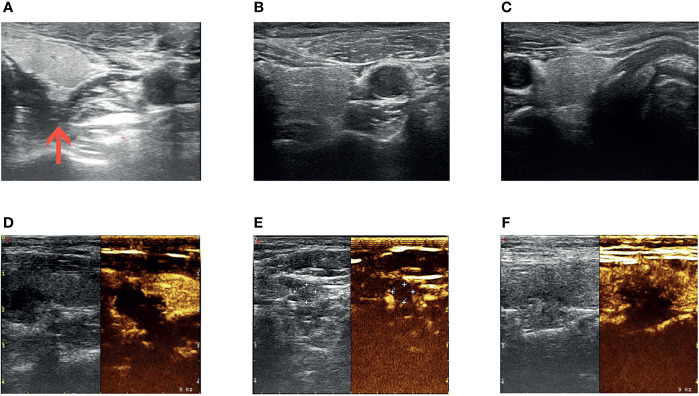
Radiofrequency ablation of the parathyroid glands. Ultrasound images of the left superior (A), left inferior (B), and right superior (C) parathyroid glands before radiofrequency ablation. Ultrasound images (left) and ultrasound contrast images (right) of left superior (D), left inferior (E), and right superior (F) parathyroid glands after radiofrequency ablation. Red arrow in (A) shows that intraoperative saline and lidocaine mixture were used for local anesthesia and isolation of the left superior parathyroid glands and peripheral tissues.
